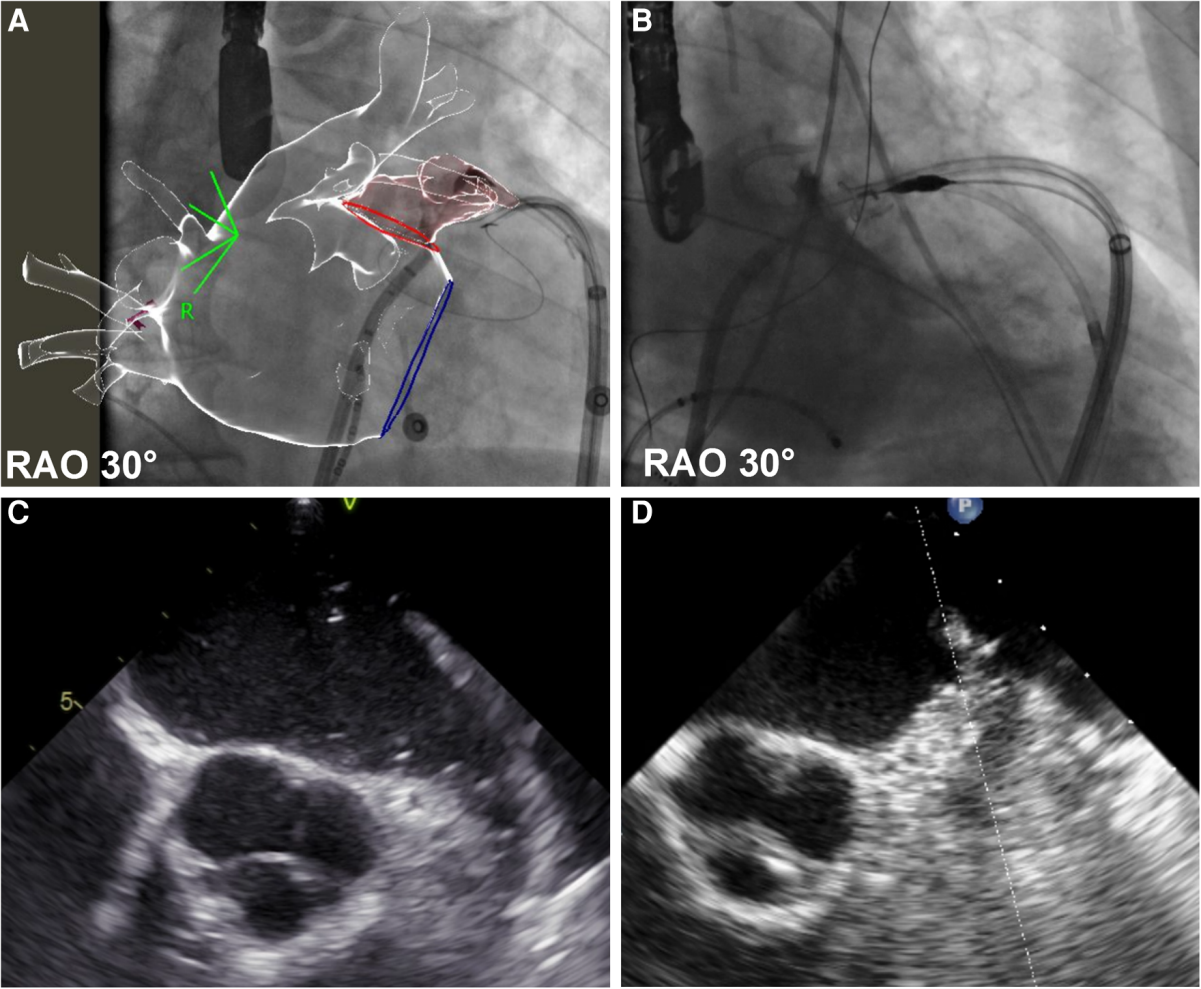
Before and after left appendage ligation. The figure shows left atrial appendage (LAA) angiography before (panel A—fluoroscopic image, panel C echocardiographic frame) and after (panel B—fluoroscopic image, panel D echocardiographic frame) LAA ligation. In panel A, fluoroscopic images are synchronized with the CT scan reconstruction, previously merged with the electro-anatomical map of the left atrium. Detailed description in text.
